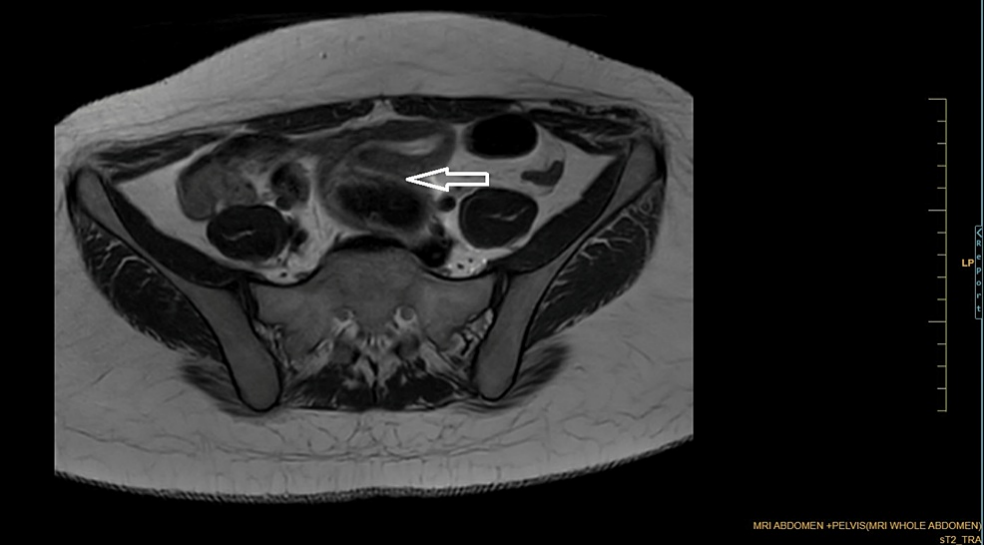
Axial T2WI showing dilated vagina with hypointense collection communicating with the left-sided uterus suggestive of hematocolpos. Note: Authors' original image T2WI: T2-weighted image
